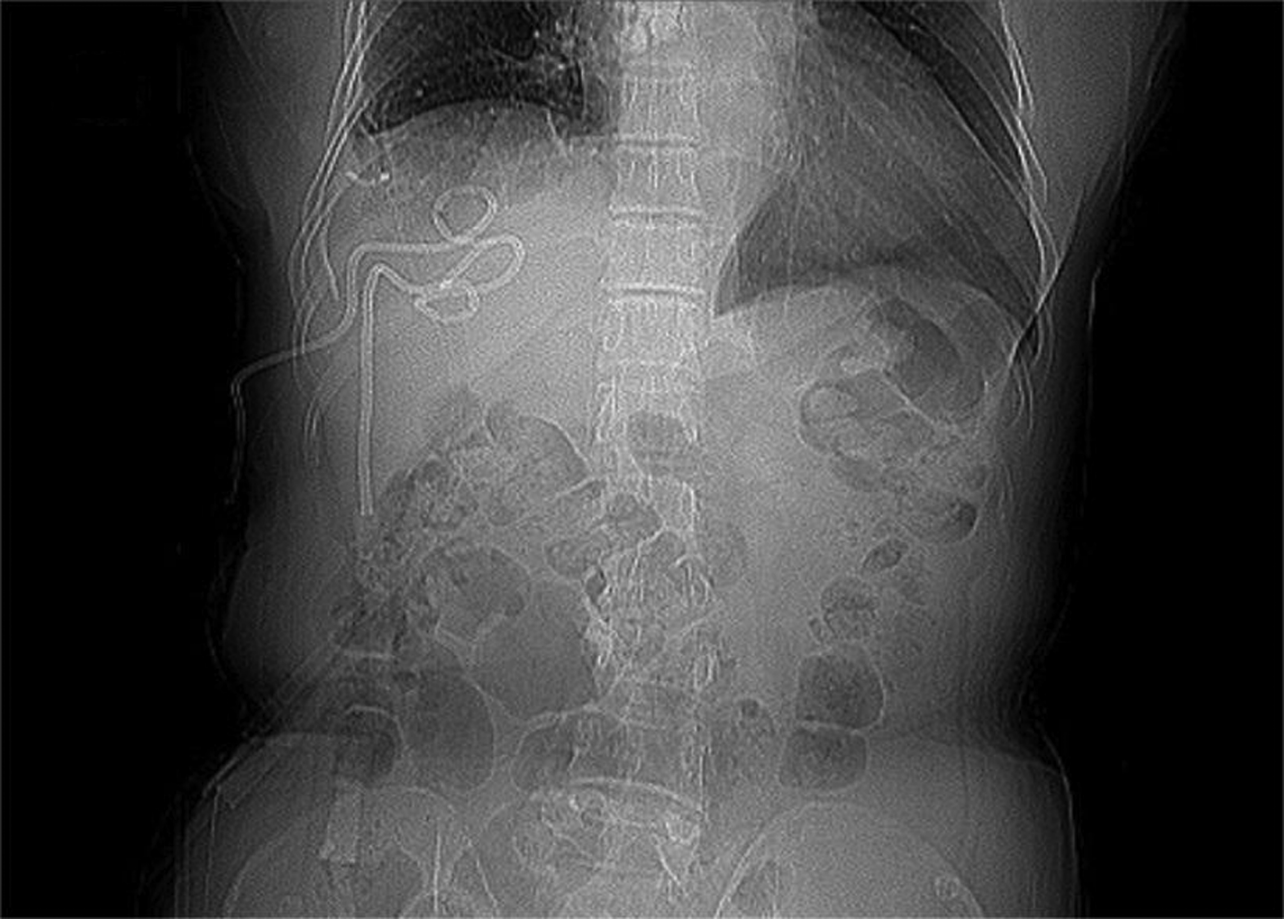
Preoperative abdominal CT suggested a large multiloculated pyogenic liver abscesses.
